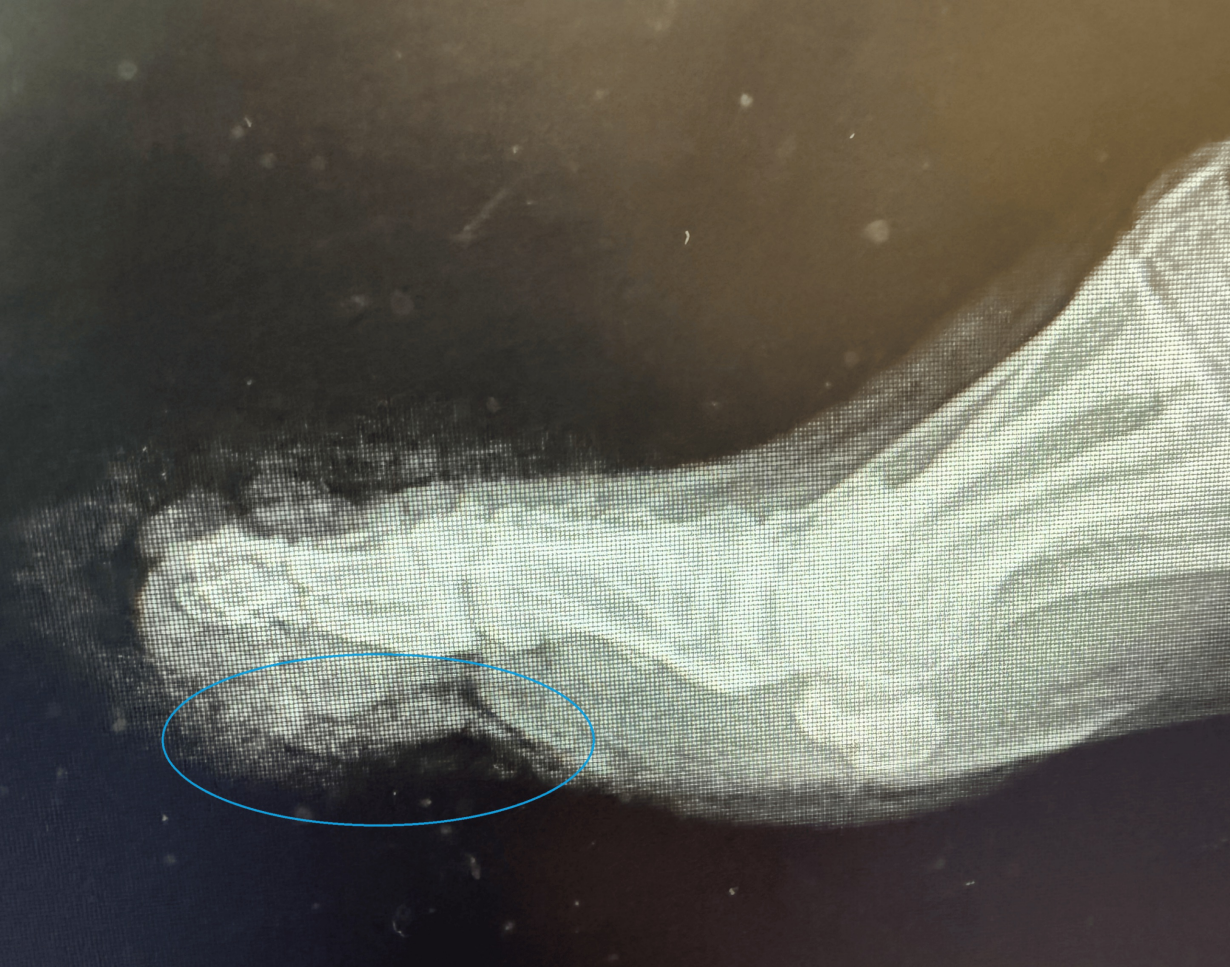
Lateral X-ray outlining extensive laceration to the patient's toes
